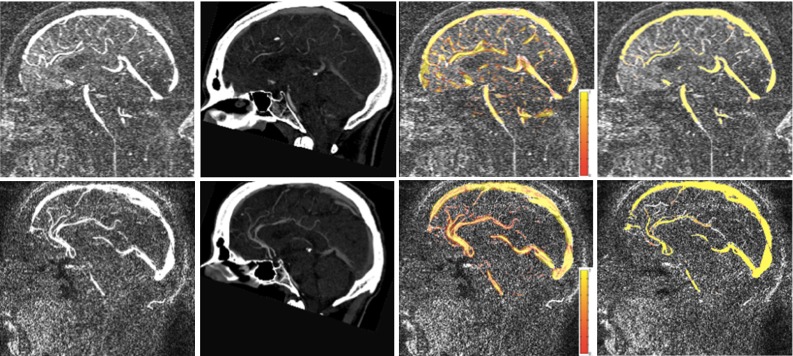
3DPC (*first column*) and CTA images (*second column*), superposed vesselness map generated by the proposed method over 3DPC (*third column*) and consensus for two subjects (*fourth column*)
